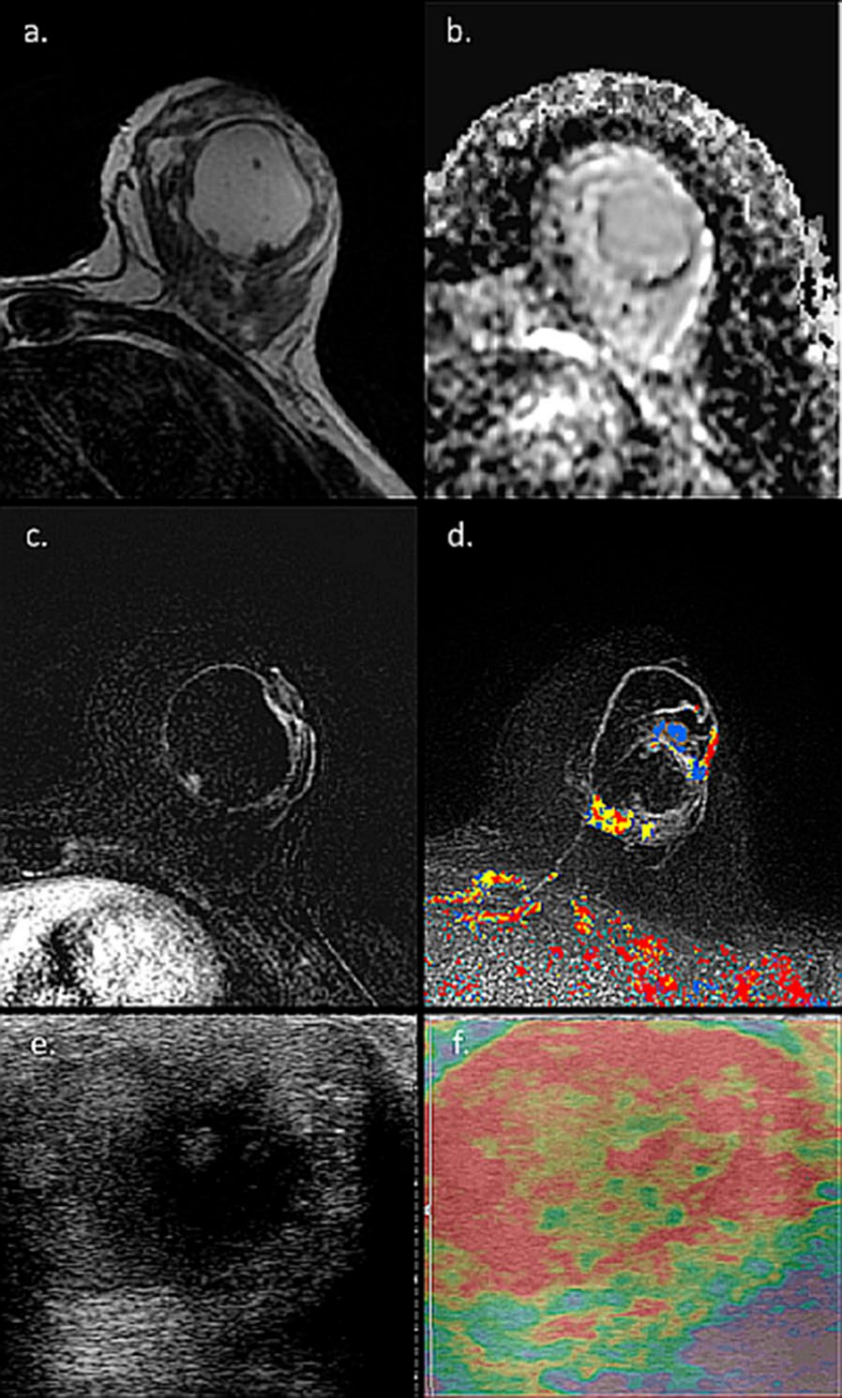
MRI of duct ectasia. MRI and US images of 40-year-old lactating patient with palpable mass on the left breast are presented. Initial work-up included US which revealed a 4 cm cystic mass with thick boundaries, with relative mixed cystic areas (e) and stiffness on static elastography (f). On MRI, T2-weighted image reveals a high signal cystic structure (a), without internal restriction on ADC map (b), though with enhancement of its walls on DCE and CAD (c, d). Finally, US-guided biopsy ruled out malignancy with duct ectasia diagnosis
